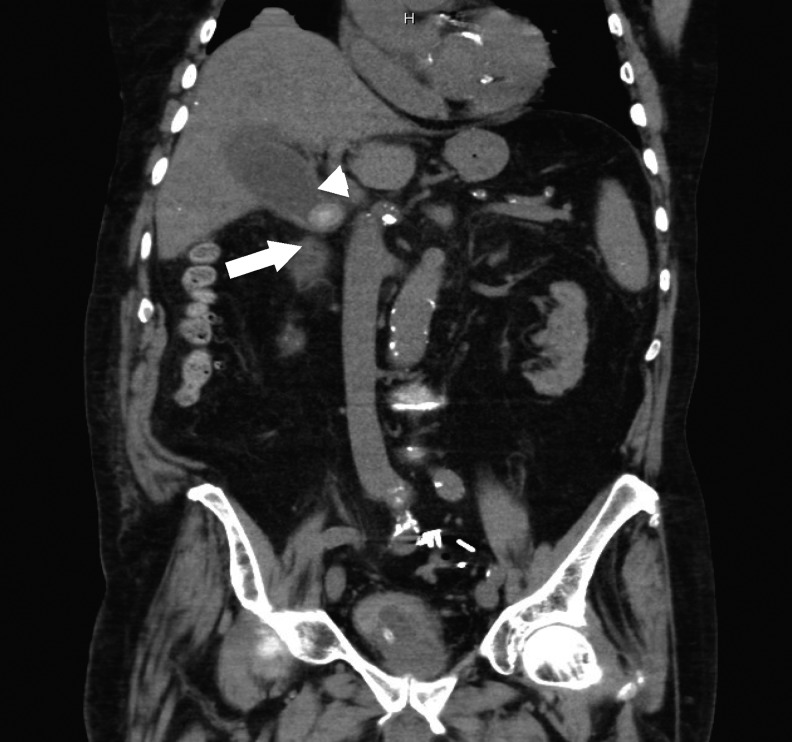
Coronal preoperative CT showing large gallstone obstructing the cystic duct (*arrow*) and pericholecytic fluid (*arrowhead*).
